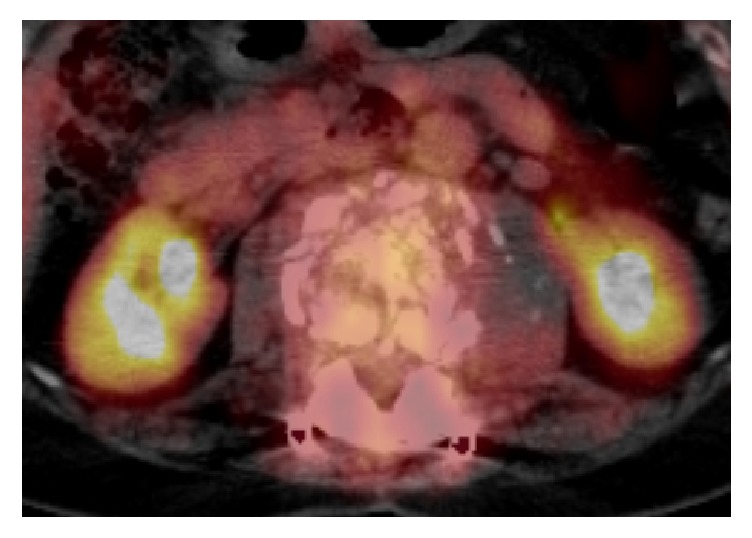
Uptake for metastases in the posterior element was not observed in PET/CT.
